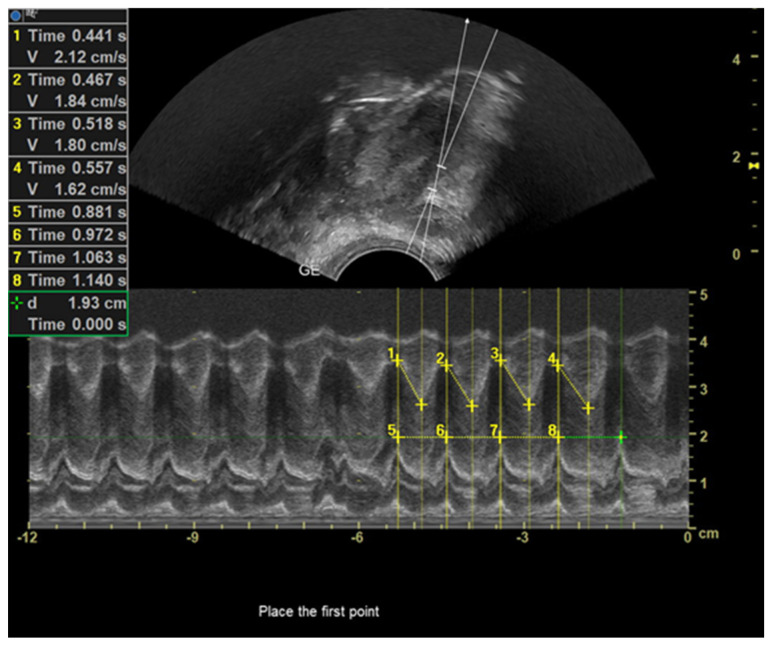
M-mode ultrasonography of tongue movement during breastfeeding. Slope measurement: yellow line 1, 2, 3 and 4; sucking cycle duration: yellow line 5, 6, 7 and 8.
